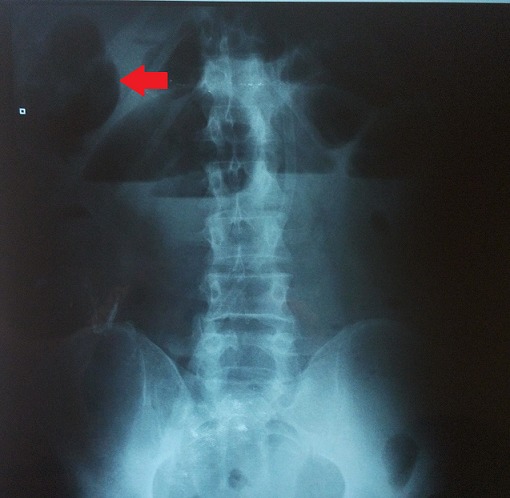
Standard radiography of the abdomen, note the small air fluid level projected upper the right quadrant
